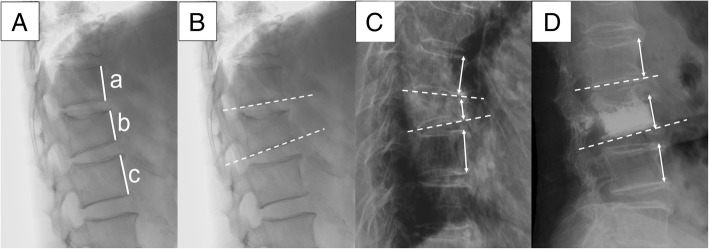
A–D Radiographic evaluation of compressed vertebrae. A Anterior vertebral height ratio (AVHR) was calculated as percentile of anterior vertebral height of the compressed vertebra (b), divided by the mean anterior vertebral height of the adjacent upper and lower vertebrae (a + c)/2. The anterior vertebral height restoration rate (AVHRR) = preoperative AVHR − postoperative AVHR. B Cobb angle was determined as the degree between the lines of lower endplate of compressed vertebra and the adjacent upper vertebra. The Cobb angle correction (CR) = preoperative Cobb angle − postoperative Cobb angle. C–D Examples of preoperative (C) and postoperative (D) images for evaluating vertebral restoration. The X-ray films were taken 1 day before and after the surgery, respectively, from an 80-year-old female patient with L3 compression fracture. Both the AVHRR (35.5% = 87.3–51.8%) and CR (11.3° = 16.1°–4.8°) are significant after treated by unilateral PKP
